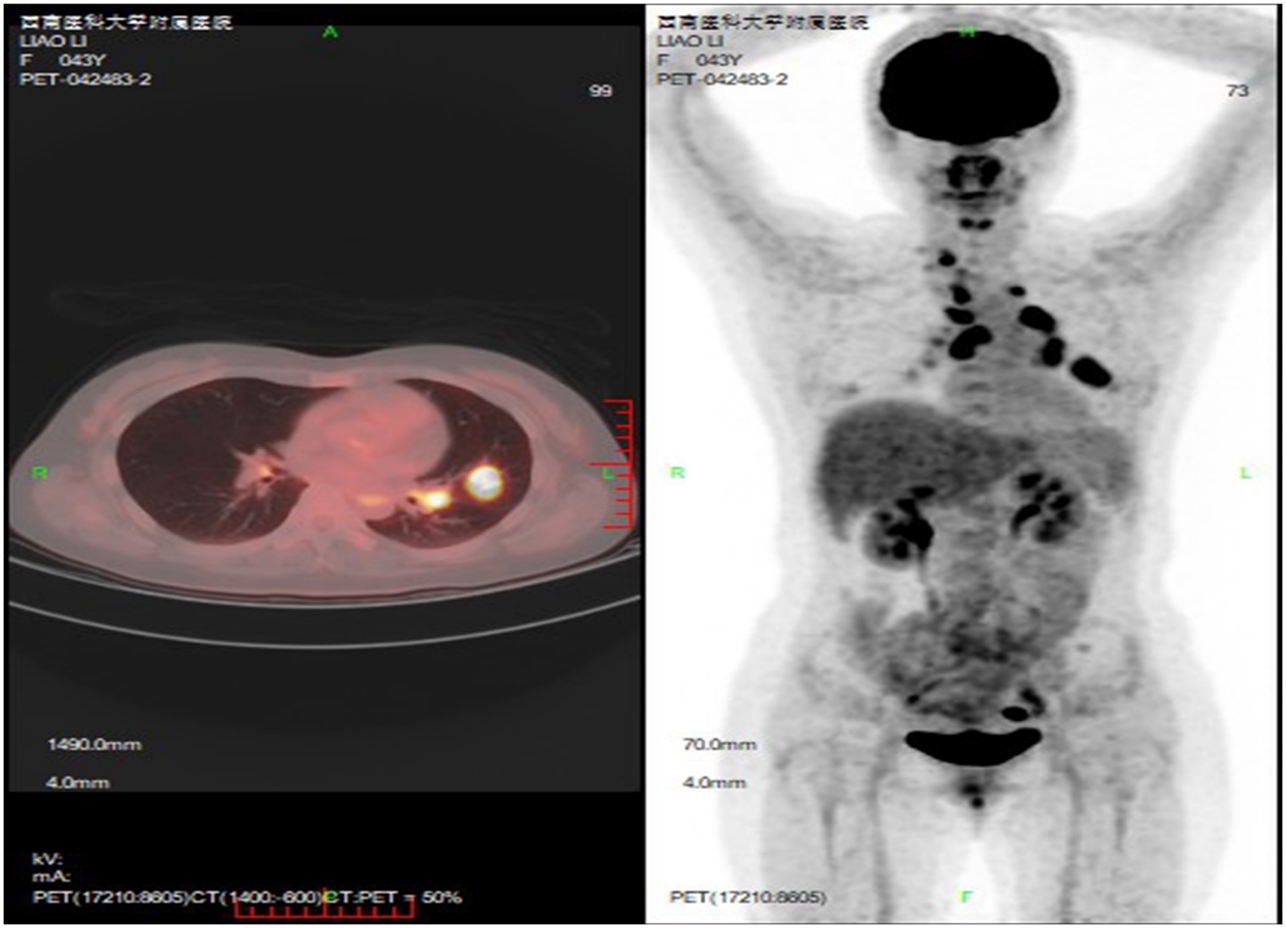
A newly developed mass was identified in the lingular segment of the left upper lobe, measuring approximately 3.6 cm × 2.8 cm in maximum cross-section, with associated increased glucose metabolism (SUVmax ≈ 8.2). Multiple enlarged hypermetabolic lymph nodes (SUVmax ≈ 12.0) were present in the right supraclavicular fossa, mediastinum, and bilateral hilar regions. There was no evidence of distant metastasis.
